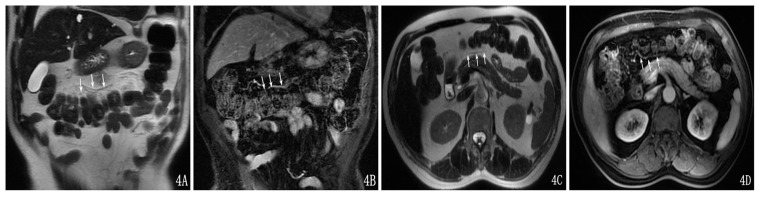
The transverse-mesocolon vessels were shown on the coronal SSFSE T2-weighted image (A), enhancement on the coronal image (B), on the axial SSFSE T2-weighted image (C) and enhancement on the axial image (D).
